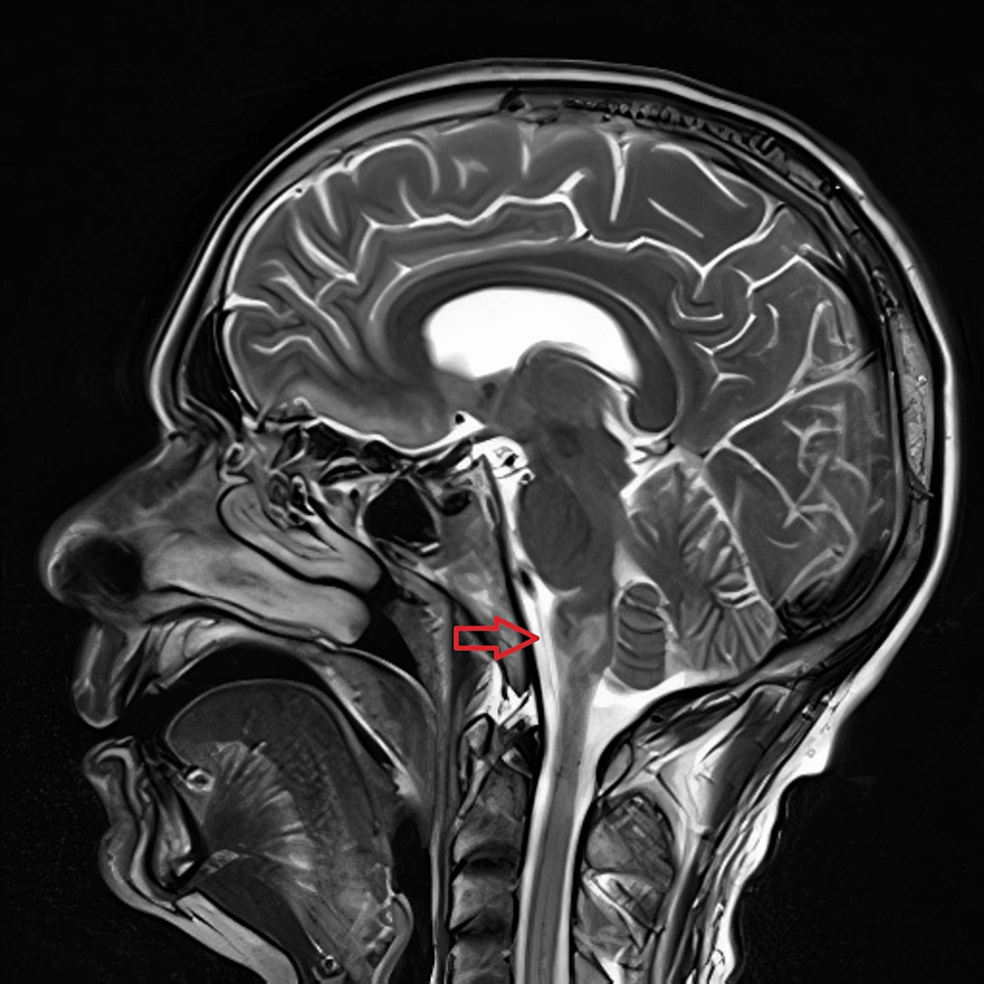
Sagittal T2-weighted brain MRI with posterior circulation infarct in the right medulla oblongata
